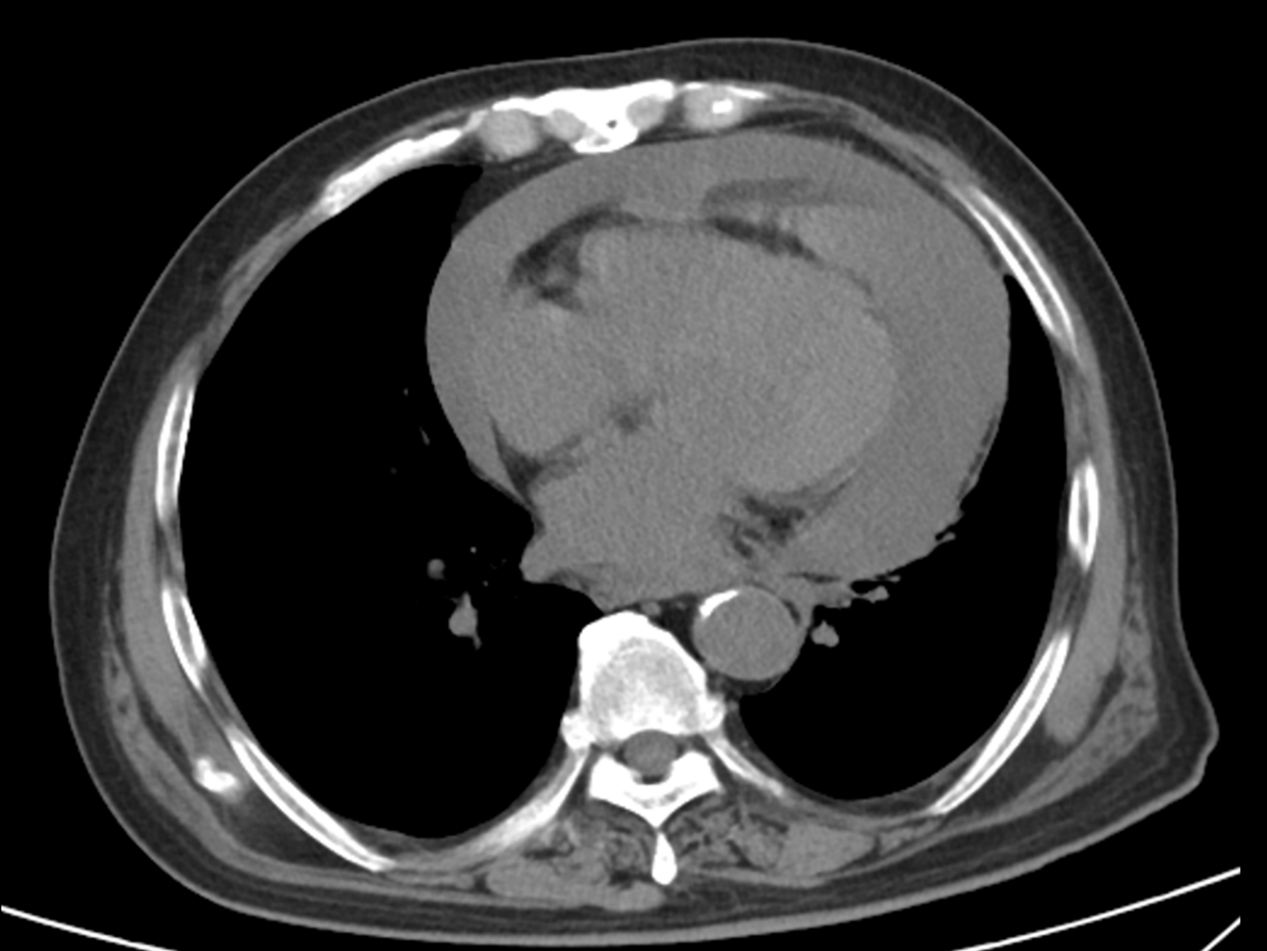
A plain CT scan of the chest (mediastinal window) on admission indicated a large pericardial effusion.
